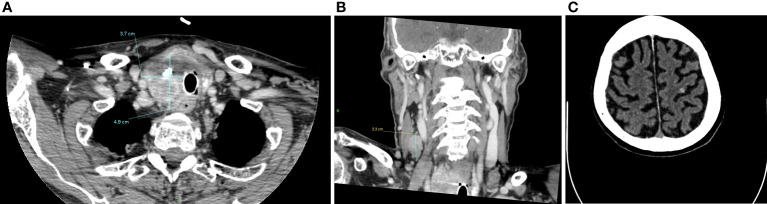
CT-scan performed in August 2021, after lenvatinib discontinuation, showing a dimensional increase of left thyroid lobe (4,9x3,7 cm) (A), latero-cervical lymphadenopathies (maximum diameter of 3 cm) (B) and multiple brain and cerebellar metastases (C).
